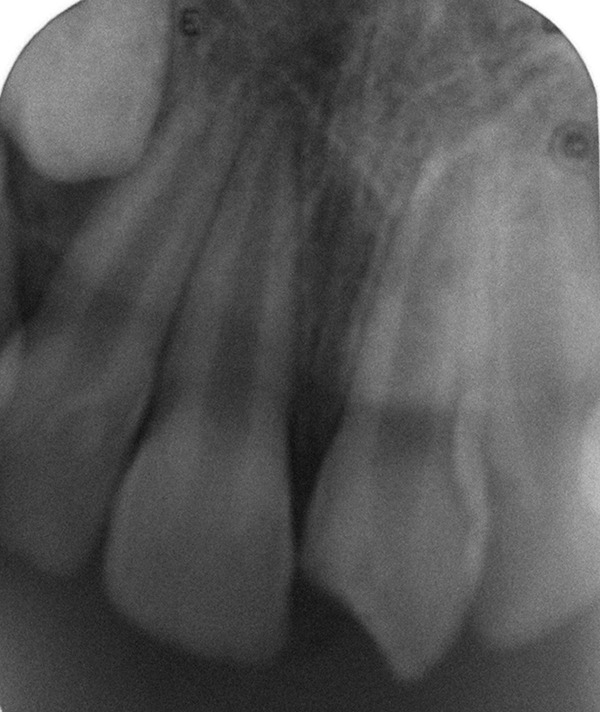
Preoperative radiograph showing oblique fracture line and arrested apical root development
